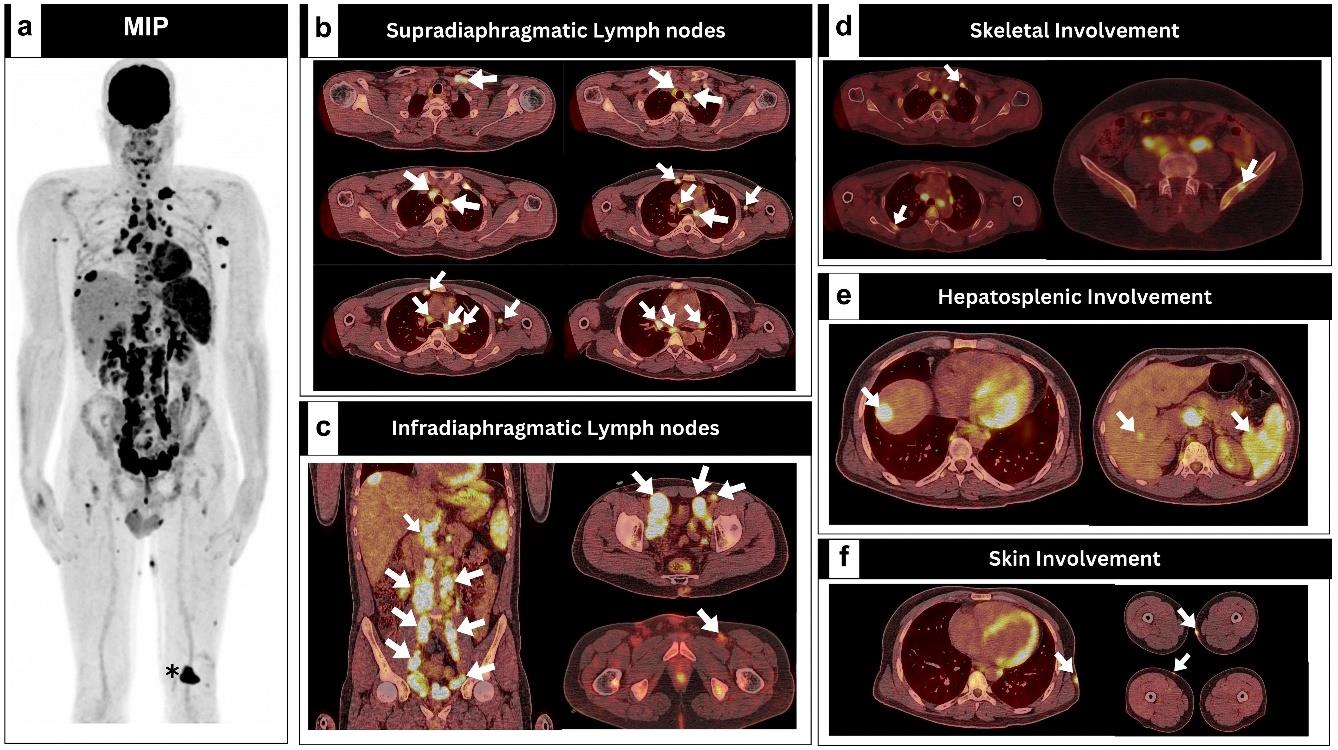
Baseline [^18^F] FDG PET/CT images demonstrating the lymphomatous disease extent (arrows) in conjunction to irrelevant single intraarticular 4 cm hypermetabolic mass lesion occupying the anteromedial aspect of the left knee (asterisk)
